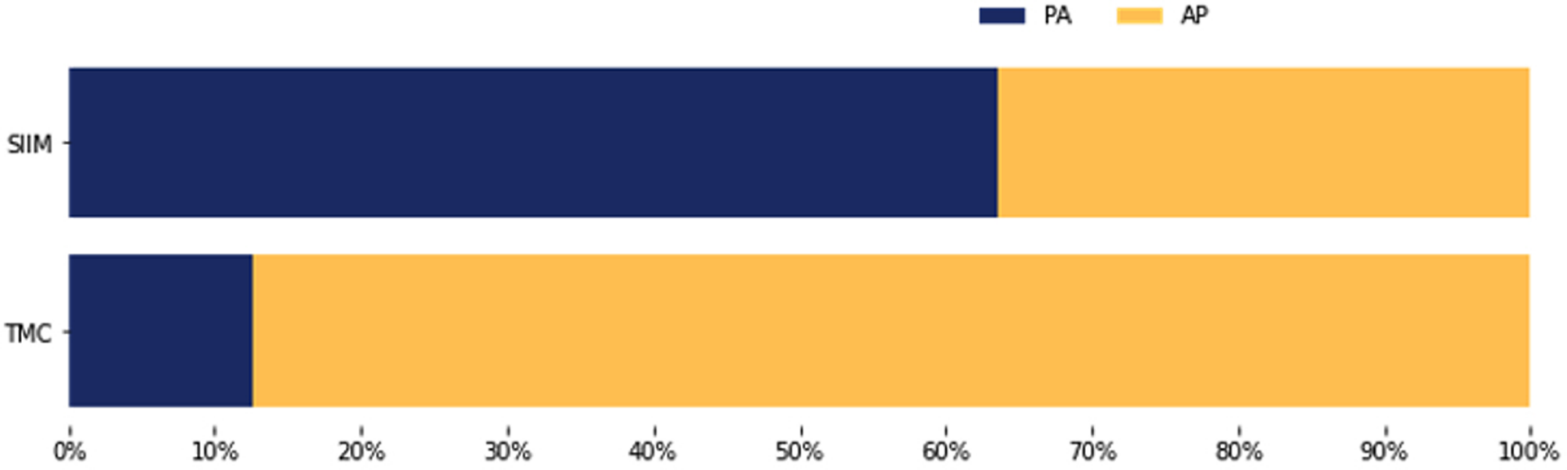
Projection of the chest X-rays diagnosed with pneumothorax in the SIIM and TMC datasets.
